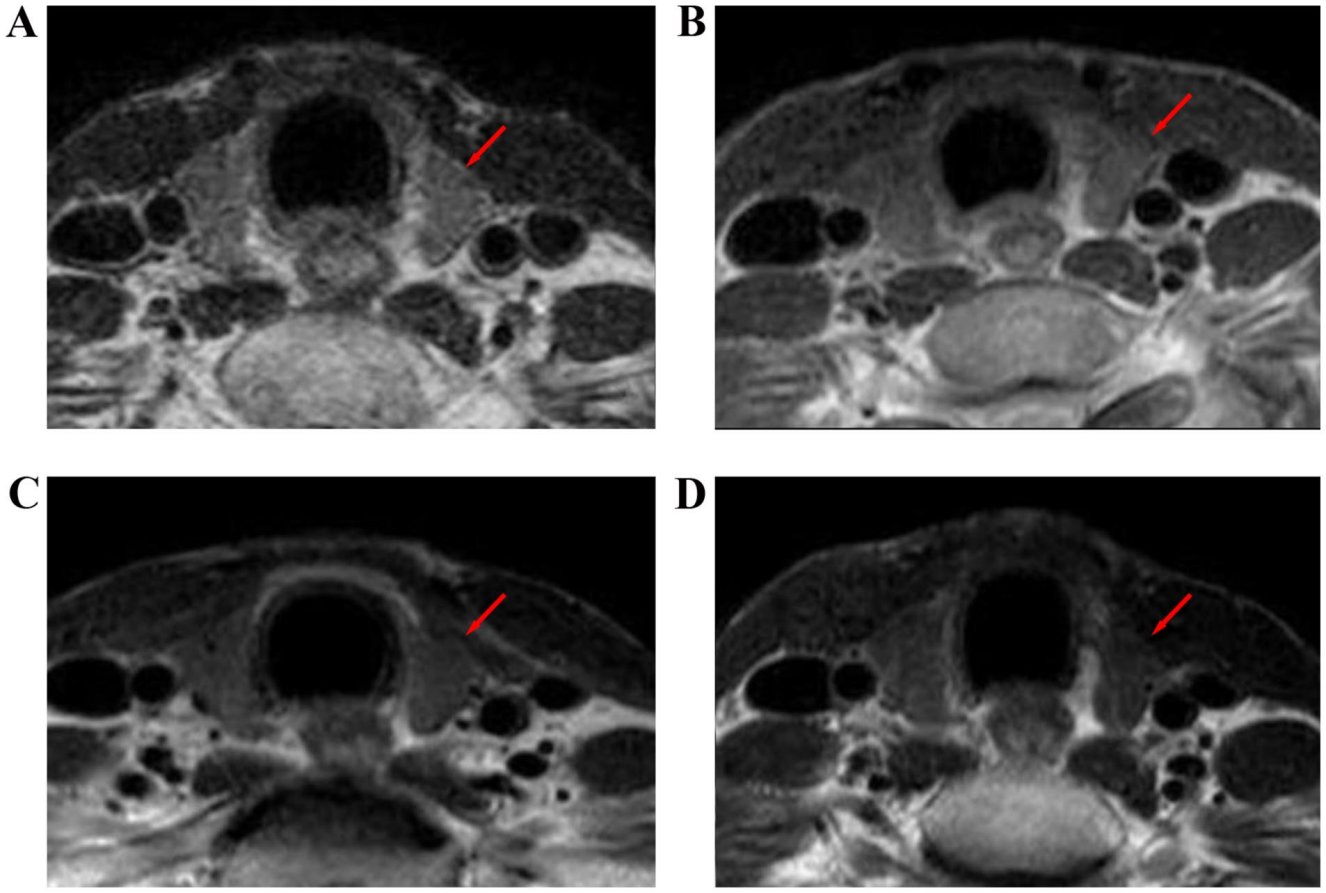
T2-weighted images of the thyroid gland before and after radiotherapy for nasopharyngeal carcinoma. (A) Before radiotherapy, (B) during radiotherapy, (C) 6 months after radiotherapy, and (D) 12 months after radiotherapy.
